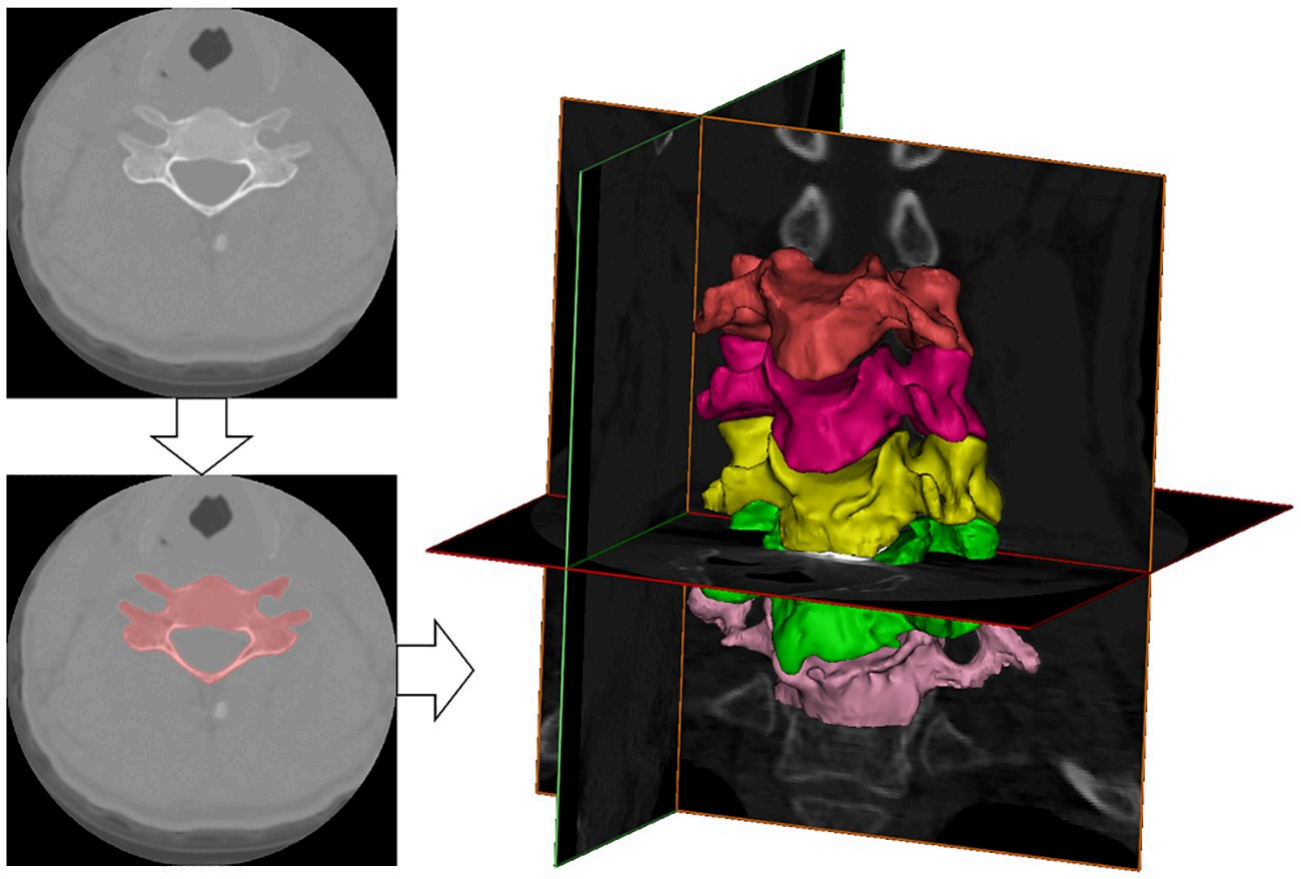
Construction of the motion model. A CT image of the cervical spine (top) is segmented, a bone mask is created (bottom; shaded area), and solid bone models were created for C4-C7 (right) using the volumetric mask, which was then used in model-based tracking.
